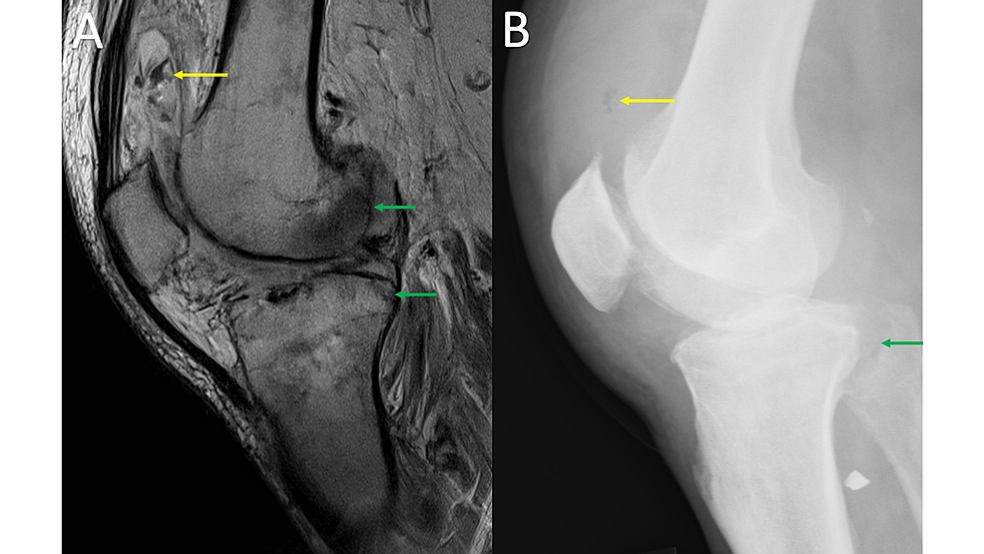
Septic Arthritis MRI sagittal proton density (PD) of the right knee (6A) shows thickened edematous synovium, subcutaneous edema, and large suprapatellar joint effusion with low PD signal (yellow arrow) within the suprapatellar bursa. Lateral radiograph of the right knee (6B) allows for easy recognition of gas (yellow arrow) within the suprapatellar bursa. Of note, there is also an abnormal bone marrow signal in the distal femur and proximal tibia (green arrows) with a radiograph showing cortical destruction involving the posterior tibia adjacent to the proximal tib-fib joint (green arrow). The final diagnosis was septic arthritis and osteomyelitis.
